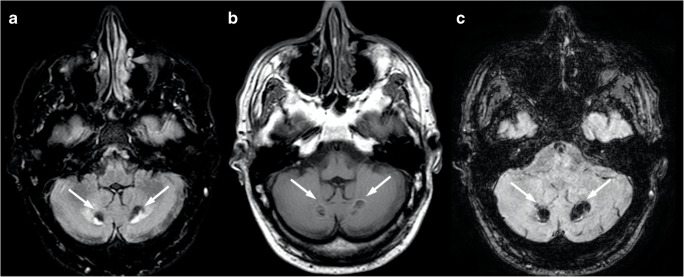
Brain MRI findings in a 42-year-old male CTX patient. Along with a mild global cerebellar atrophy, it is present gliosis and calcifications of the deep cerebellar WM, extending to the peri-dentate region (arrows), as shown in the axial FLAIR (a), T1-weighted (b), and SWI (c) sequences
